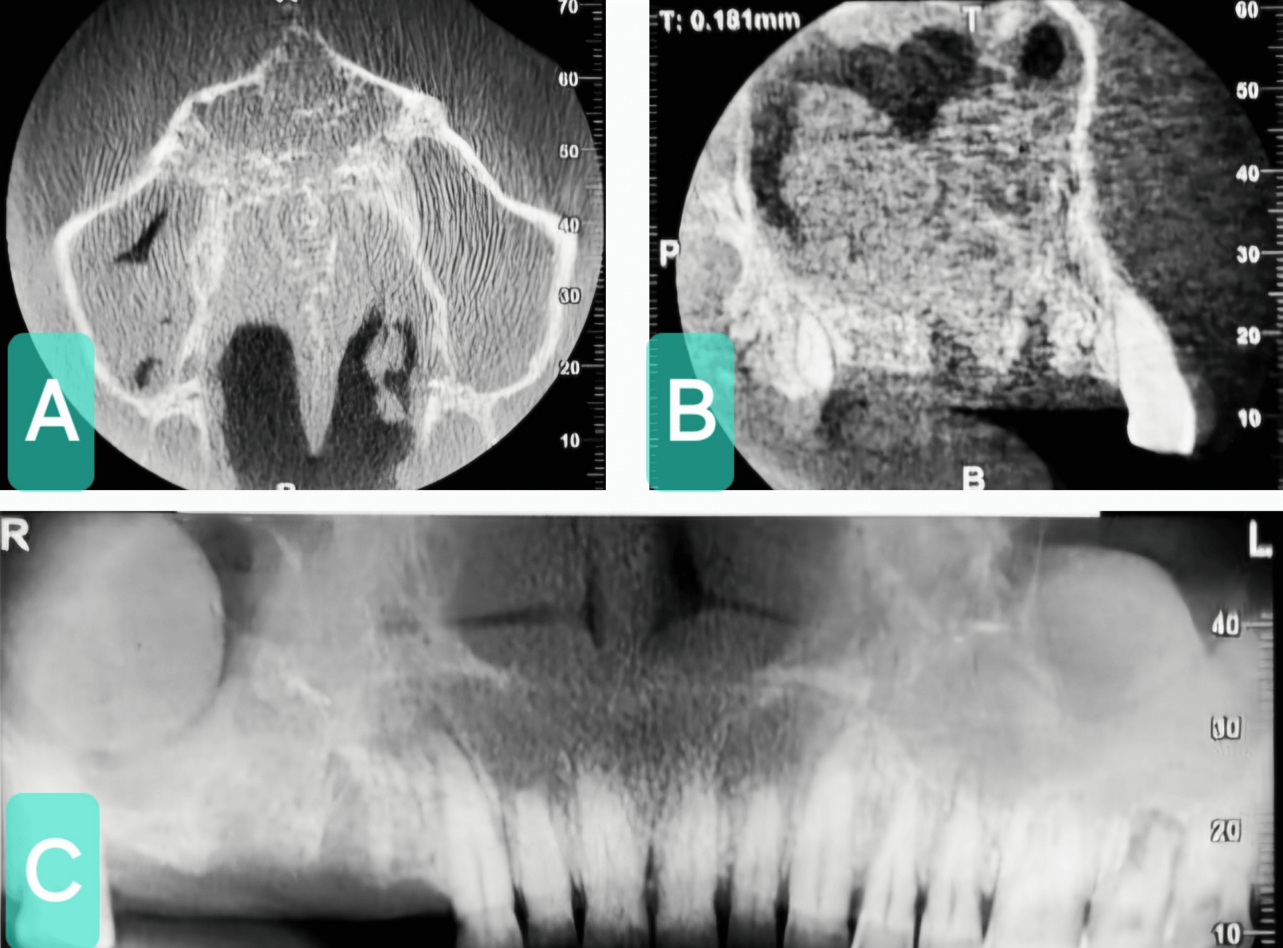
Cone-bean CT scan. (A) Axial view. (B) Sagittal view. (C) A well-defined soft tissue mass can be seen within the maxillary antrum.
